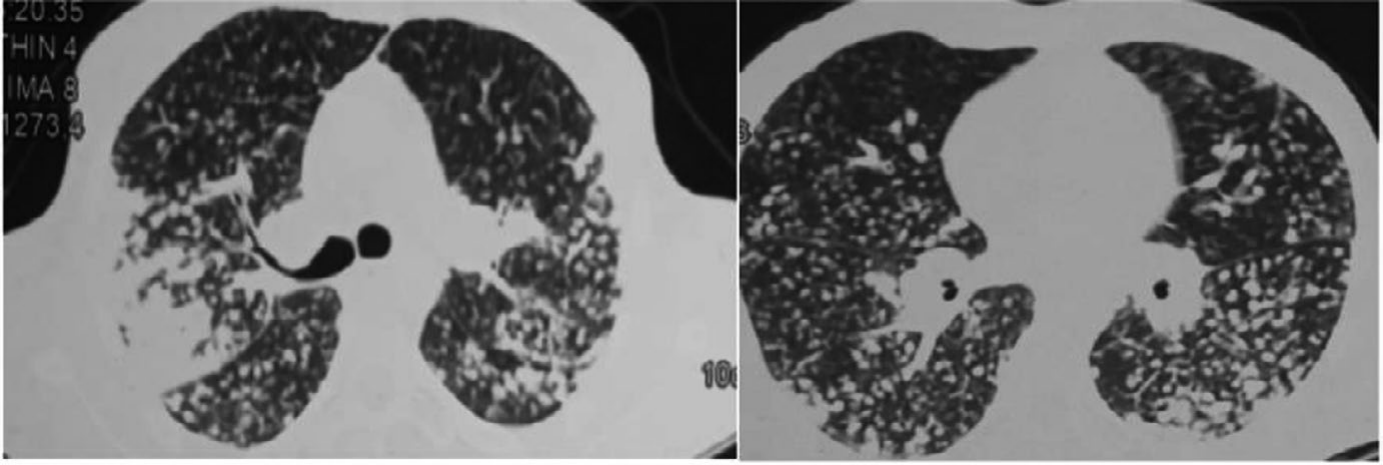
Chest CT scan of patient 2 shows multiple pulmonary nodules with random distribution and condensation of the upper right lobe
